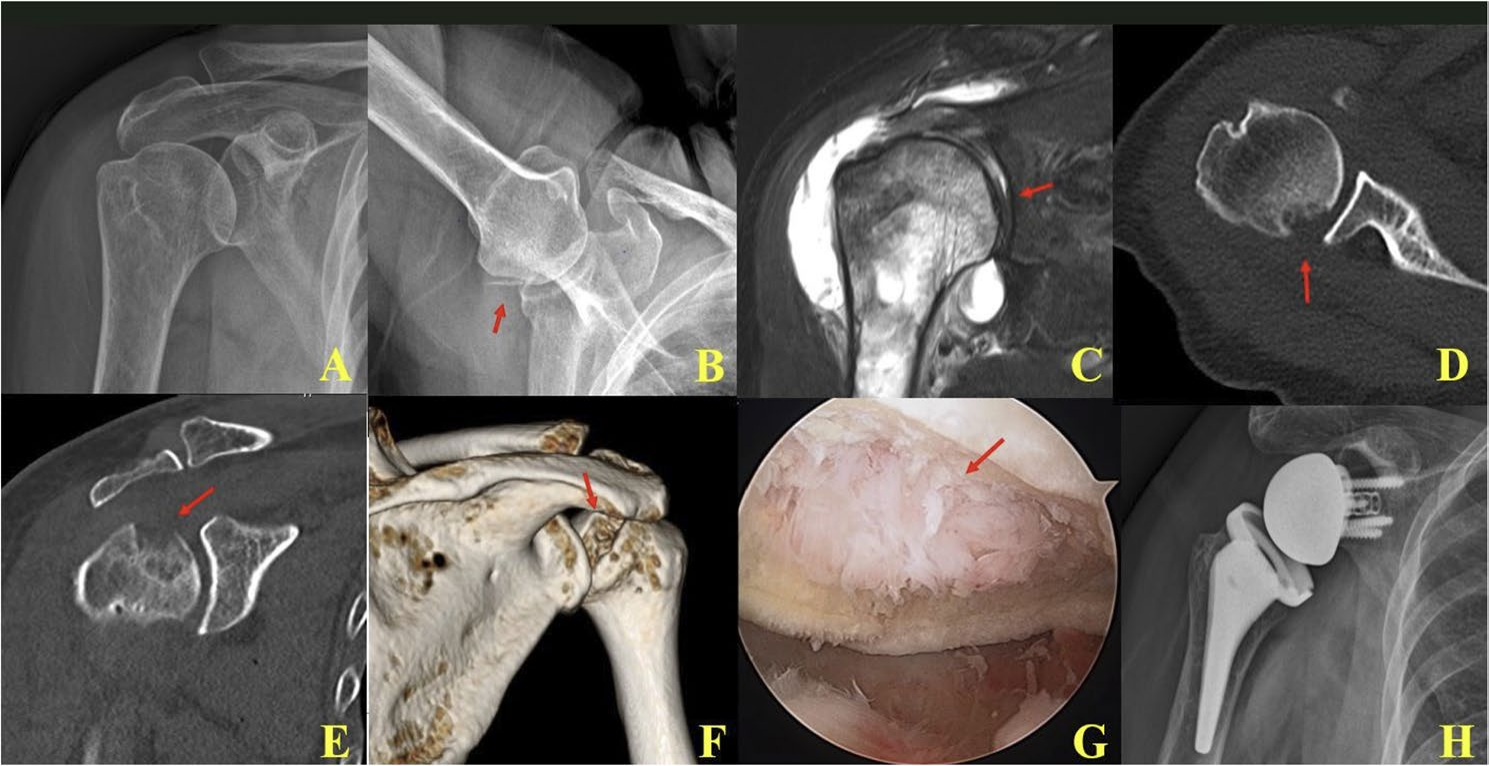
In the early prodromal phase of RPDA: A, B Initial plain radiographs demonstrated no abnormalities; however, follow-up imaging at six months revealed a subchondral fracture in the posterolateral aspect of the humeral head. C Initial MRI showed partial bone marrow edema of the humeral head, rather than diffuse involvement. D, E Follow-up CT scans at six months clearly demonstrated a subchondral fracture. (F) 3D CT revealed posterolateral collapse of the humeral head. G Arthroscopic evaluation showed complete loss of articular cartilage, embedded fibrotic tissue, and synovial hypertrophy. H Due to persistent shoulder pain, reverse total shoulder arthroplasty was performed one month later
